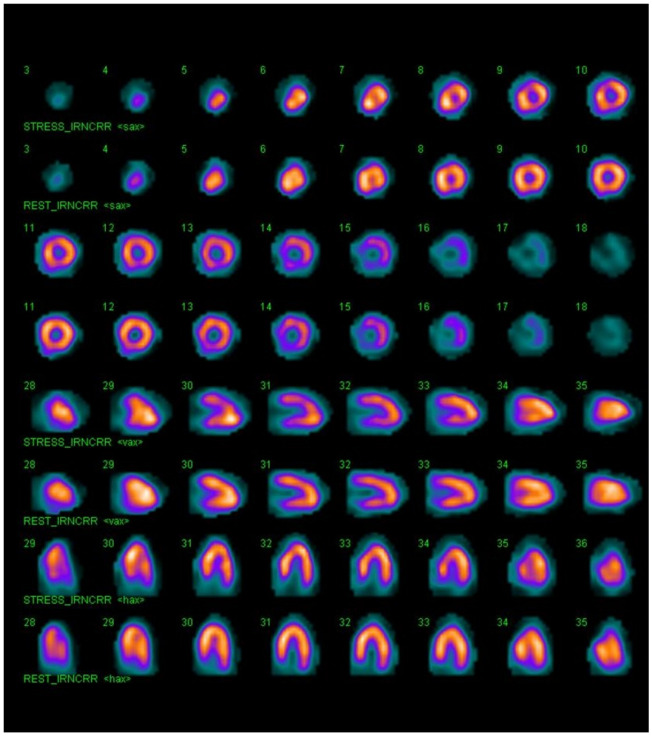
A 59-year-old male patient with complete muscle bridge on the third segment of the anterior descending artery (IVA 3). Myocardial perfusion SPECT with technetium-99m tetrofosmin performed after pharmacologic stress with regadenoson and at rest demonstrates homogeneous tracer uptake throughout all myocardial segments, with no evidence of reversible or fixed perfusion defects. Left ventricular size and function are within normal limits. Ejection fraction is preserved.
